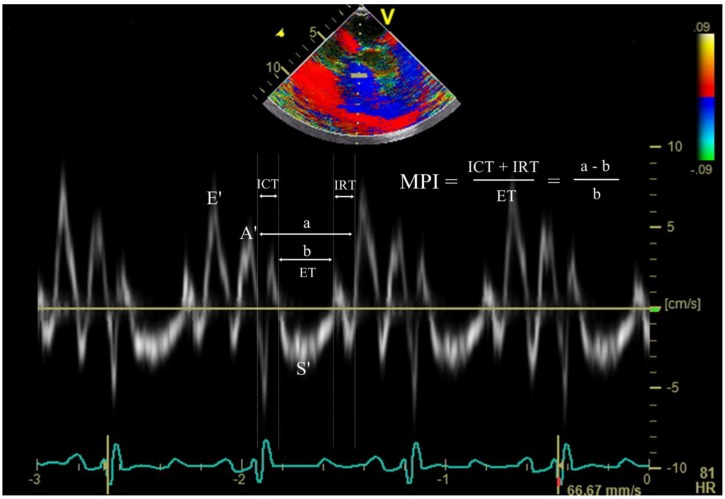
Doppler tissue waves derived from the septal mitral annulus, and time intervals required for calculation of myocardial performance index. E’, peak early diastolic mitral annular velocity; A’, late diastolic mitral annular velocity; S’, peak systolic mitral annulus velocity; ICT, isovolumic contraction time; IRT, isovolumic relaxation time; ET, ejection time; MPI, myocardial performance index.
